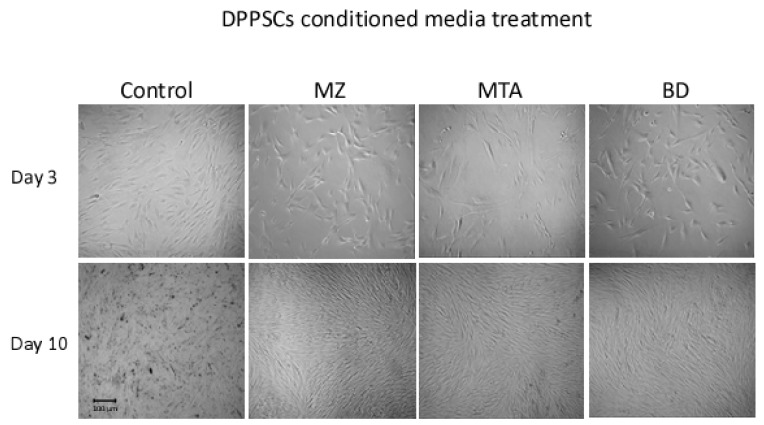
Cell morphology. Dental Pulp Pluripotent-like Stem Cells (DPPSCs) at day 3 (100×) and at day 10 (40×) of differentiation. BD, Biodentine; MZ, MedCem; MTA, Mineral Trioxide Aggregate; CT, Control media.
